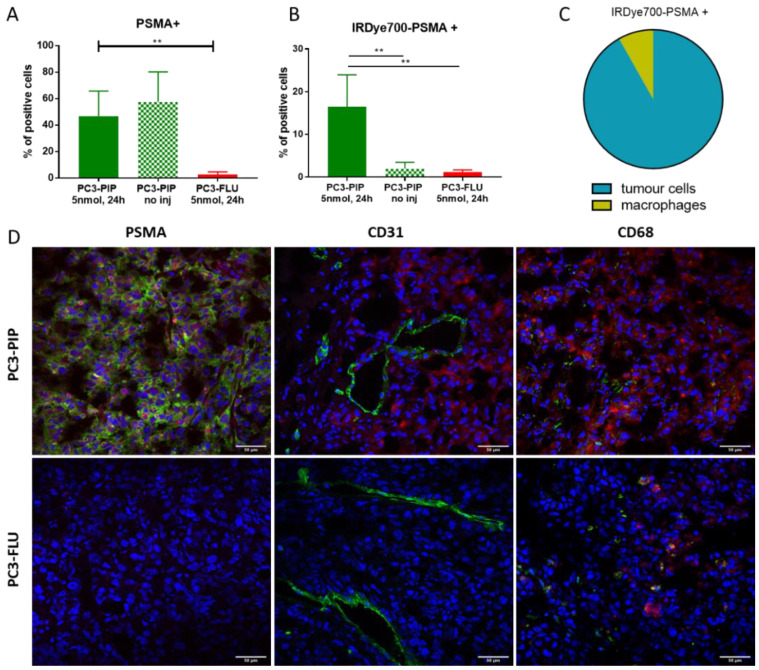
Ex vivo tumour characterization. (A) Flow cytometry data of % of PSMA positive cells in PC3-PIP and PC3-FLU tumour tissue.  *p* < 0.01, 1-way ANOVA repeated measures followed by Bonferroni post hoc test. (B) Flow cytometry data of % IRDye700DX-PSMA positive cells in PC3-PIP and PC3-FLU tumour tissue.  *p* < 0.01, 1-way ANOVA repeated measures followed by Bonferroni post hoc test (C) Flow cytometry data of IRDye700DX-PSMA cellular distribution in PC3-PIP tumours. (D) Confocal fluorescent images of PC3-PIP and PC3-FLU tumours with IRDye700DX-PSMA in red, nuclei in blue and in green PSMA, CD31 or CD68, respectively. Magnification 40×, PI (nuceli) 535–617 nm, AlexaFluor488 (PSMA, CD31, CD68) 498–577 nm, IRDye700DX 650–750.
